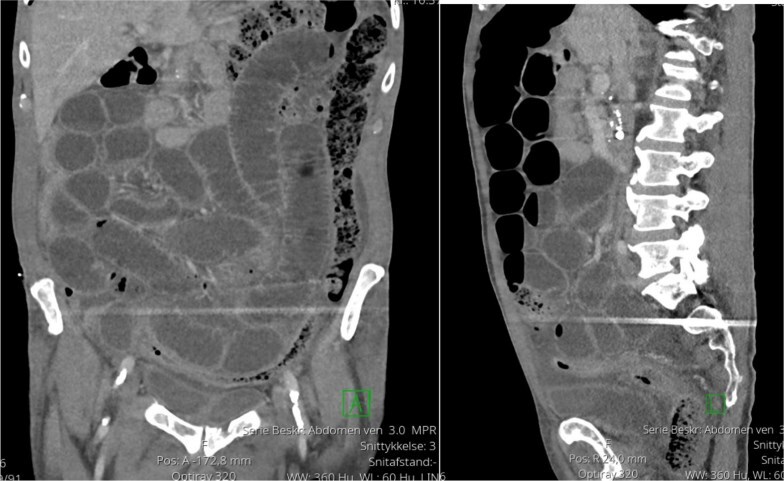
Abdominal surgery acute protocol (ASAP) CT scan of abdomen at first readmission on suspicion of intestinal obstruction.
